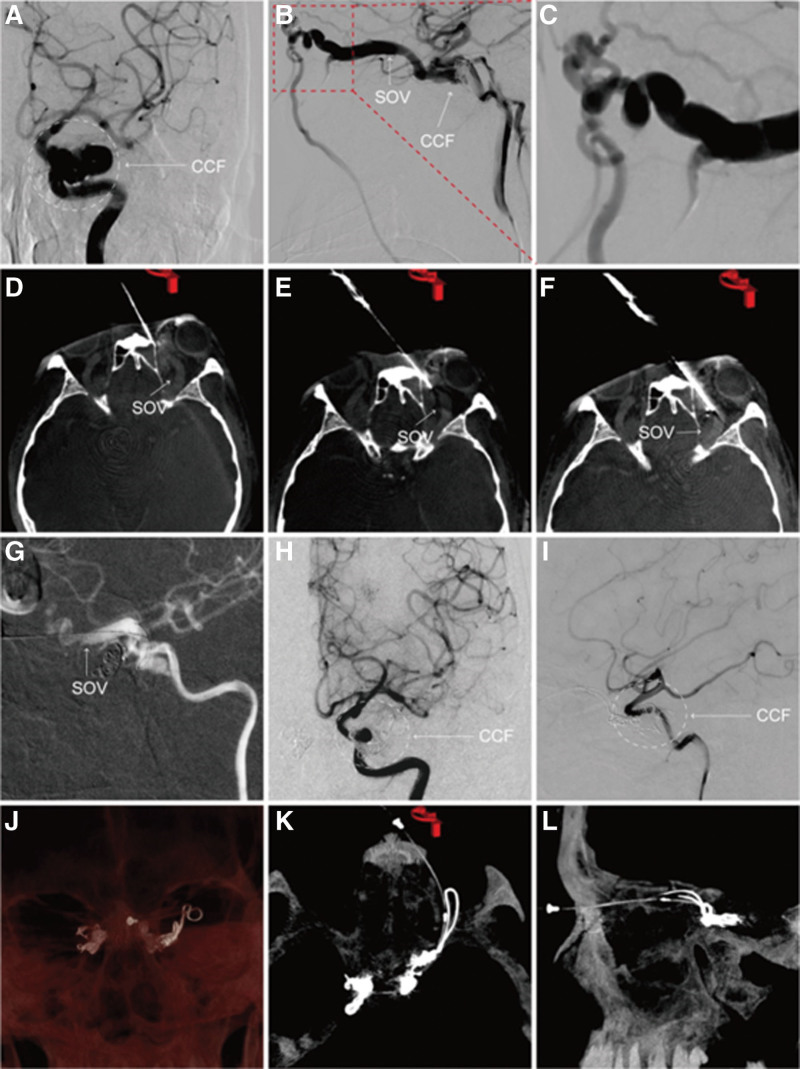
(a−b) DSA images showed that the left TCCF and the left SOV were remarkably dilated. (c) The AV was constituted with tortuous vessels. (d−f) The process of puncture the SOV guided by Dyna-CT. (g) After successful puncture the SOV, super-selective the CS and obliterated the fistula with coils. (h) and (i) Angiography images showed the fistula was completely disappearing. (j−l) Dyna-CT images were reconstructed and showed the coils in the bilateral TCCF. AV = angular vein, CS = cavernous sinuses, DSA = digital subtraction angiography, SOV = superior ophthalmic vein, TCCF = traumatic carotid-cavernous sinus fistula.
